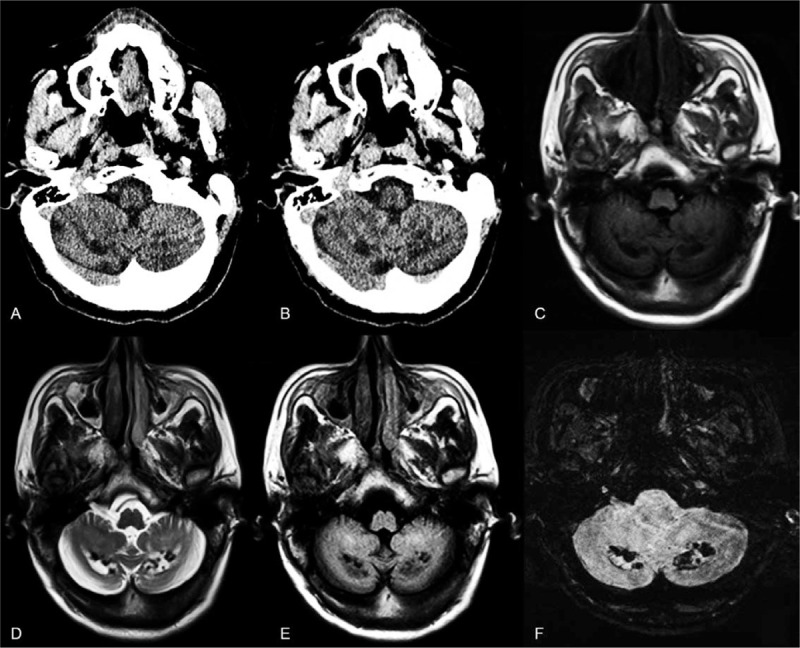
Cerebellar parenchymal abnormalities in the patient. (A) and (B) Axial CT scans show low density in both cerebellar hemispheres. (C) Axial T1W images show hypointensities in both dentate nuclei and bilateral cerebellar deep white matter. (D) Axial T2W images show hyperintensities in the bilateral cerebellar deep white matter with low-intensity areas in the dentate nuclei. (E) Axial FLAIR image shows low signal intensity consistent with cerebellar vacuolation in the same areas. (F) SWI reveals hypointensities in the bilateral dentate nuclei compatible with microcalcifications. CT = computed tomography, FLAIR = fluid-attenuated inversion recovery, SWI = susceptibility-weighted imaging, T1W = T1-weighted, T2W = T2-weighted.
